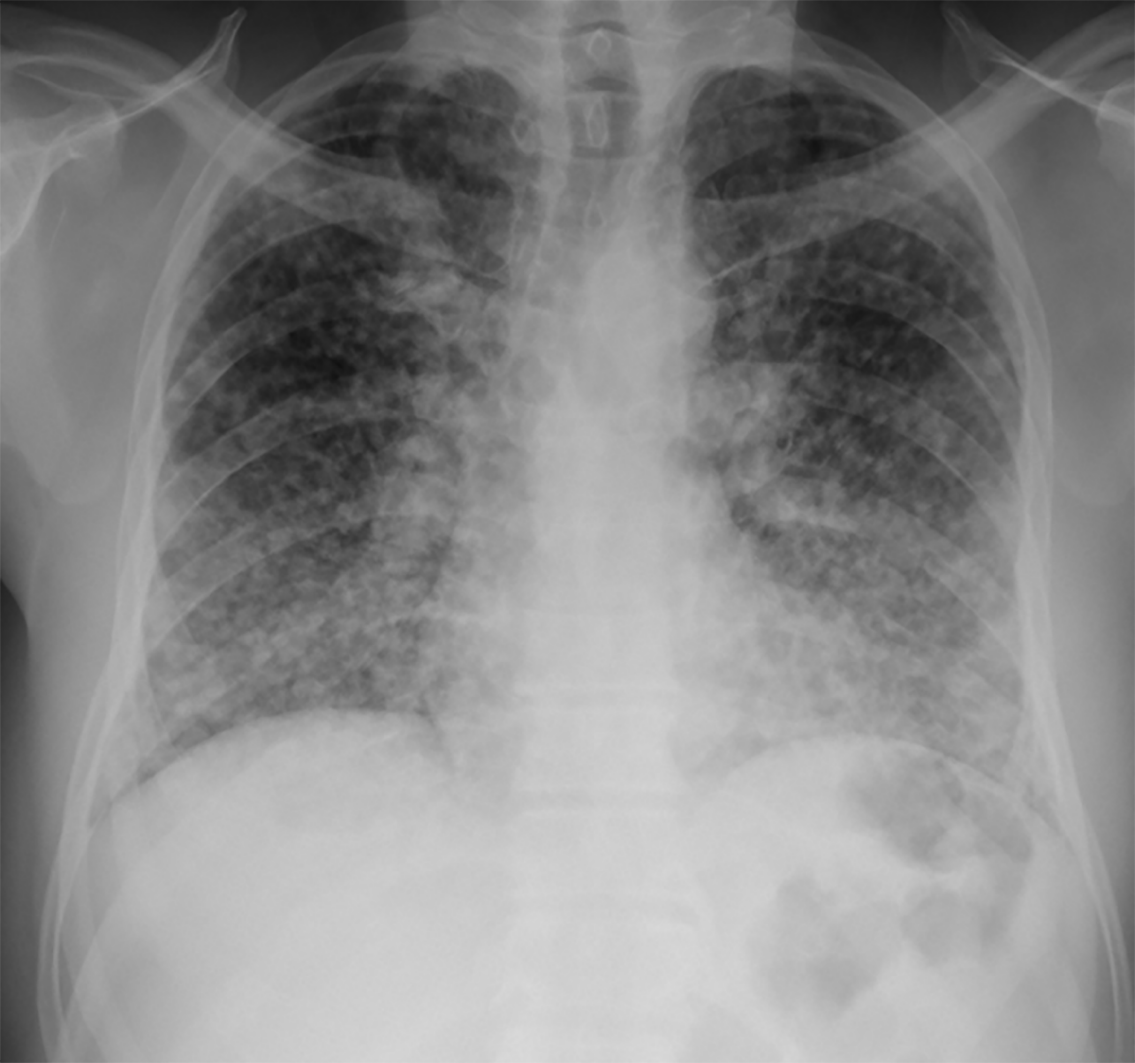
A 55-year-old man with no previous comorbidities presented with a 3-week history of cough, expectoration, fatigue, fever, night sweats, dyspnoea, and weight loss. A chest X-ray showed a miliary pattern. The patient was diagnosed with histoplasmosis after lung biopsy and positive serology.
